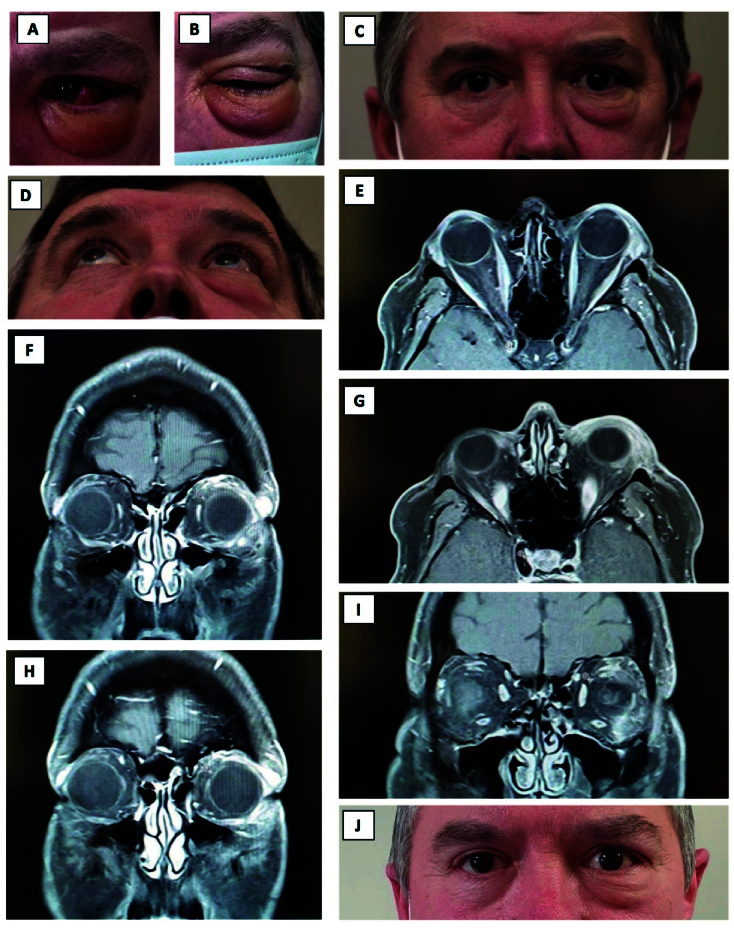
Case 1 (57-year-old male). Photographs showing left lower lid swelling (A–C). T1 weighted MRI head and orbit, axial (E, G) and coronal sections (F, H, I) showing an enlarged lacrimal gland with some ill-defined edges, enhancement, and thickening in the anterior orbit, extending inferotemporal and along the inferior orbital rim, but not involving recti. Photograph taken one-month post intralesional steroid injection (J).
